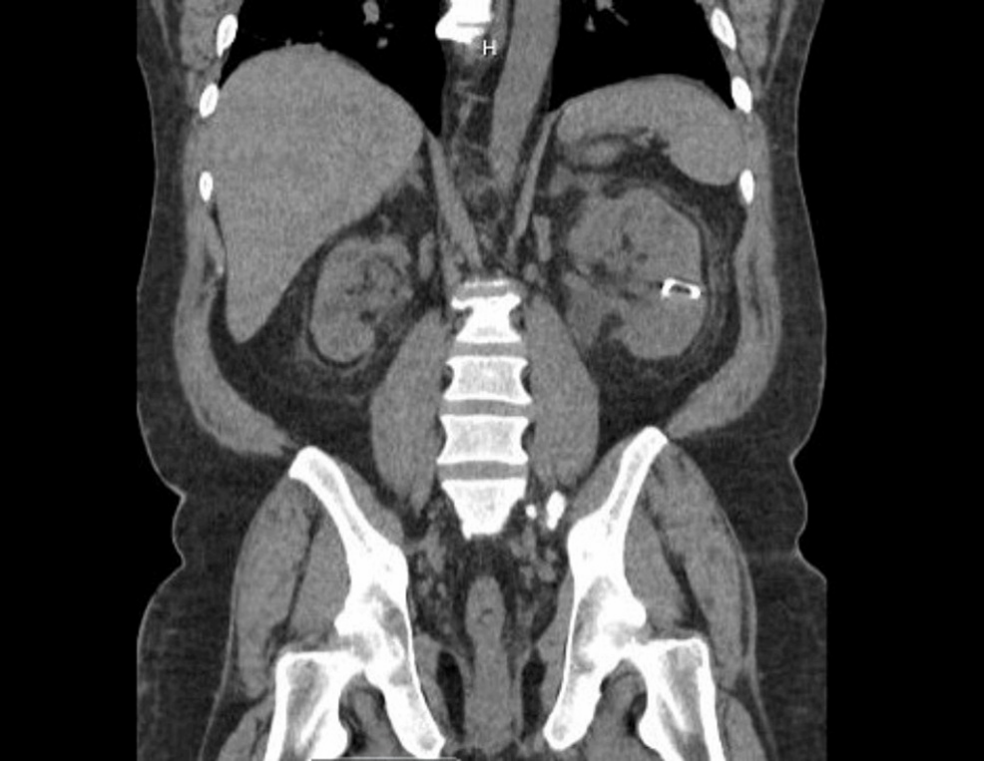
Coronal view on CT showing improved hydronephrosis with residual patulous ureters, with urinary bladder now positioned within the pelvis. The left percutaneous nephrostomy tube appears just outside of the calyx.
